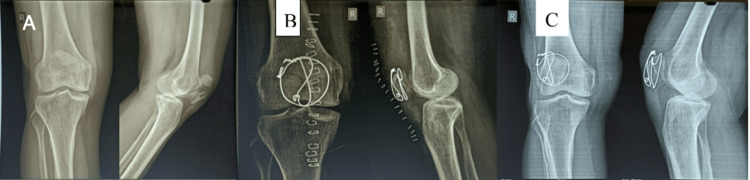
(A) Preoperative X-rays. (B) Immediate postoperative X-rays. (C) Follow-up X-rays at 16 weeks
